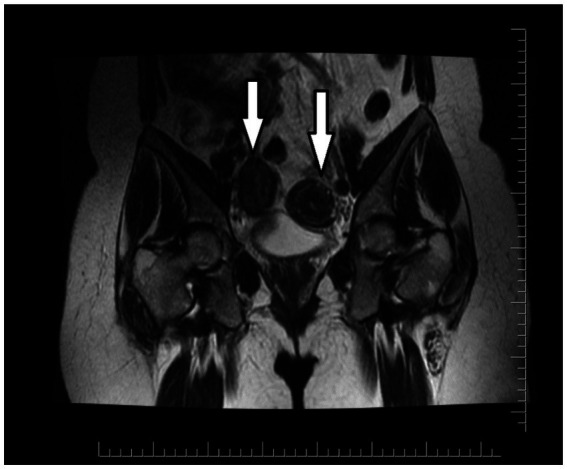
MRI: Coronal T2-weighted image shows the presence of a normal didelphic uterus at the 6-month follow-up (white arrows).
